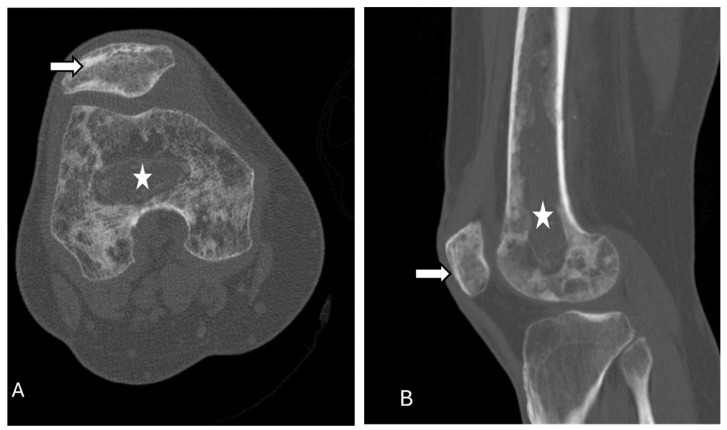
Osteomyelitis. Axial (A) and sagittal (B) CT scans of knee show diffusely osteopenic femur and patella with a moth-eaten appearance (arrows), likely secondary to osteomyelitis healing changes and disuse. There is also another area of focal osteopenia adjacent to the antibiotic beads (stars) within the femoral medullary cavity. The images were obtained from the University of Washington Medical Imaging database with the required permissions.
